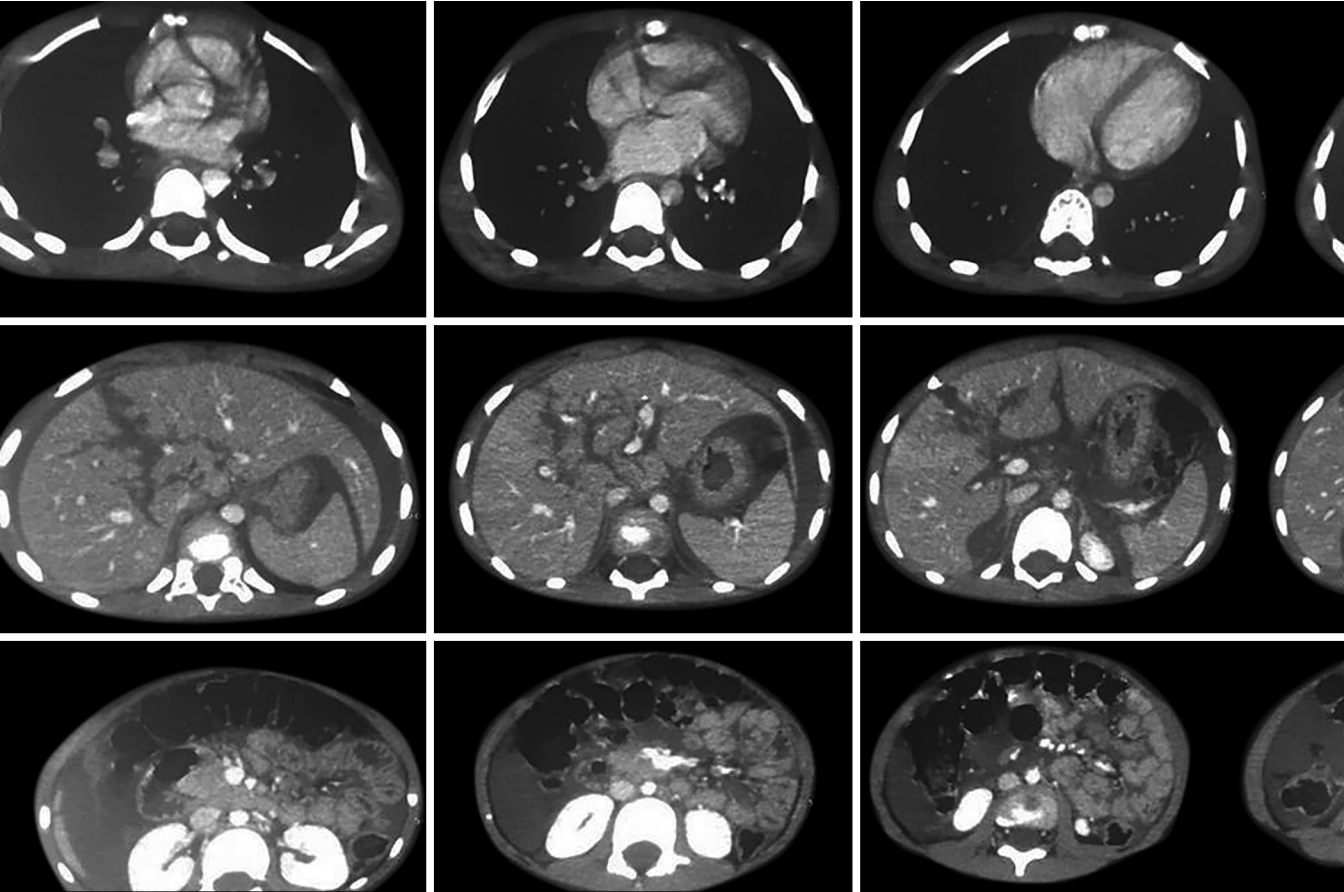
Contrast-enhanced computed tomography of abdomen showing massive hemoperitoneum with AAST grade IV trauma in segments III and IV of liver and AAST grade I trauma in spleen
